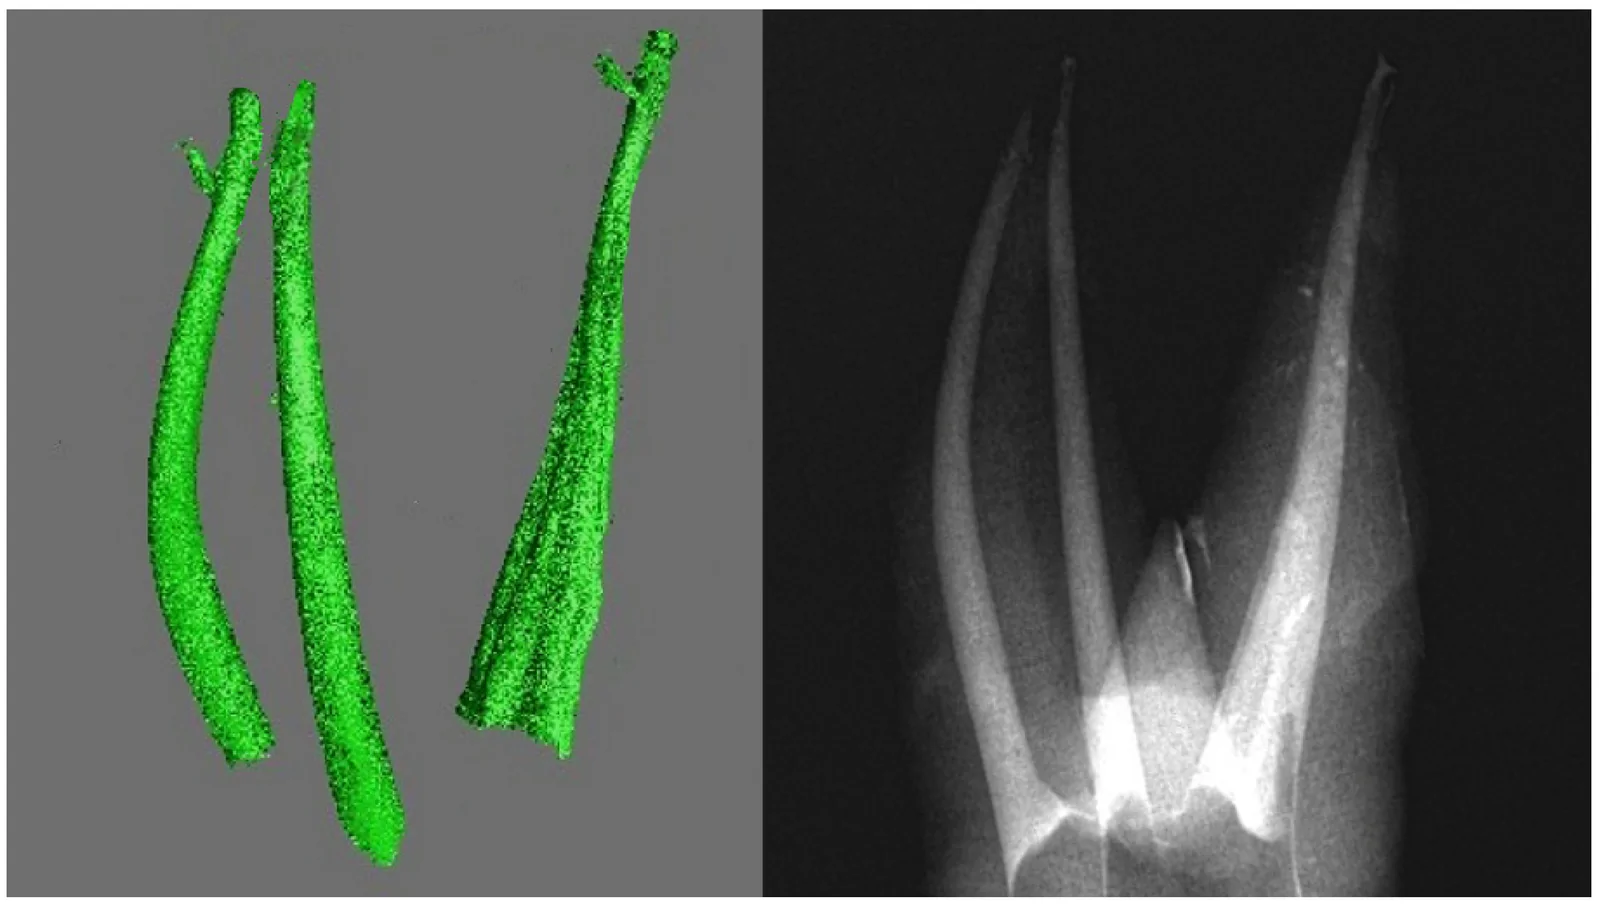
CT and radiographic image of the root canal filling.
